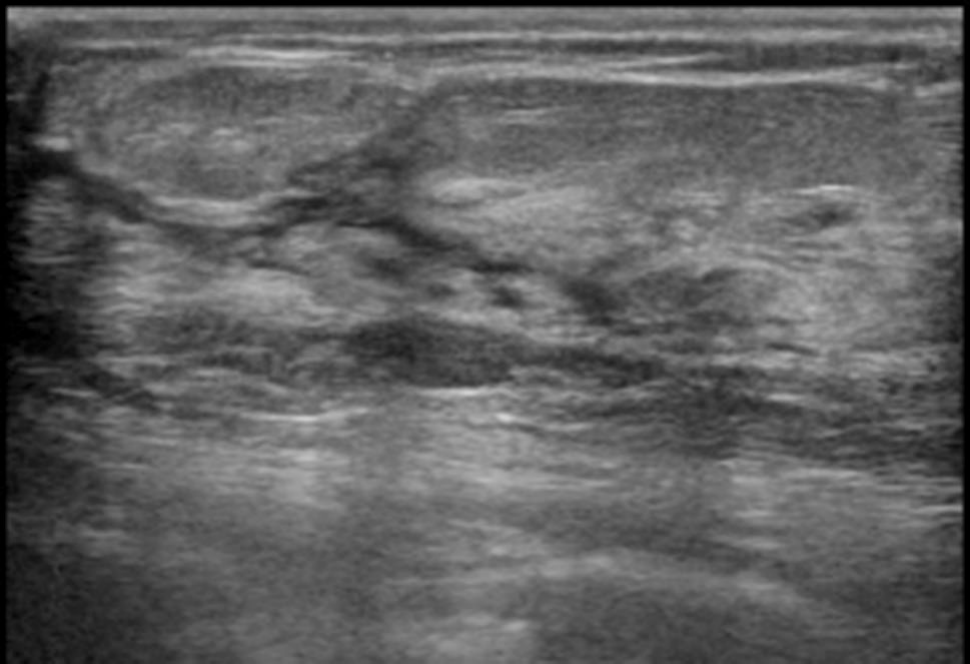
Case of ductal carcinoma in situ (DCIS). Ultrasound shows the irregularity of ductal caliber, but it is somewhat difficult to evaluate irregularity of ductal caliber based on static images alone
